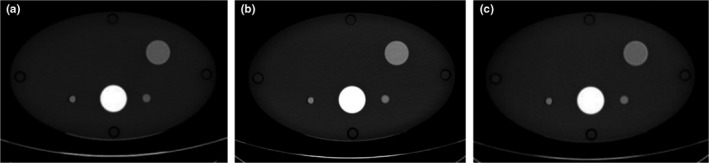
Example of images used for measuring enhancement in positively enhancing lesions (a) true unenhanced image (b) 70‐keV monochromatic image reconstructed from the Dual‐Energy post‐contrast dataset (c) virtual unenhanced image reconstructed from Dual‐Energy computed tomography dataset.
